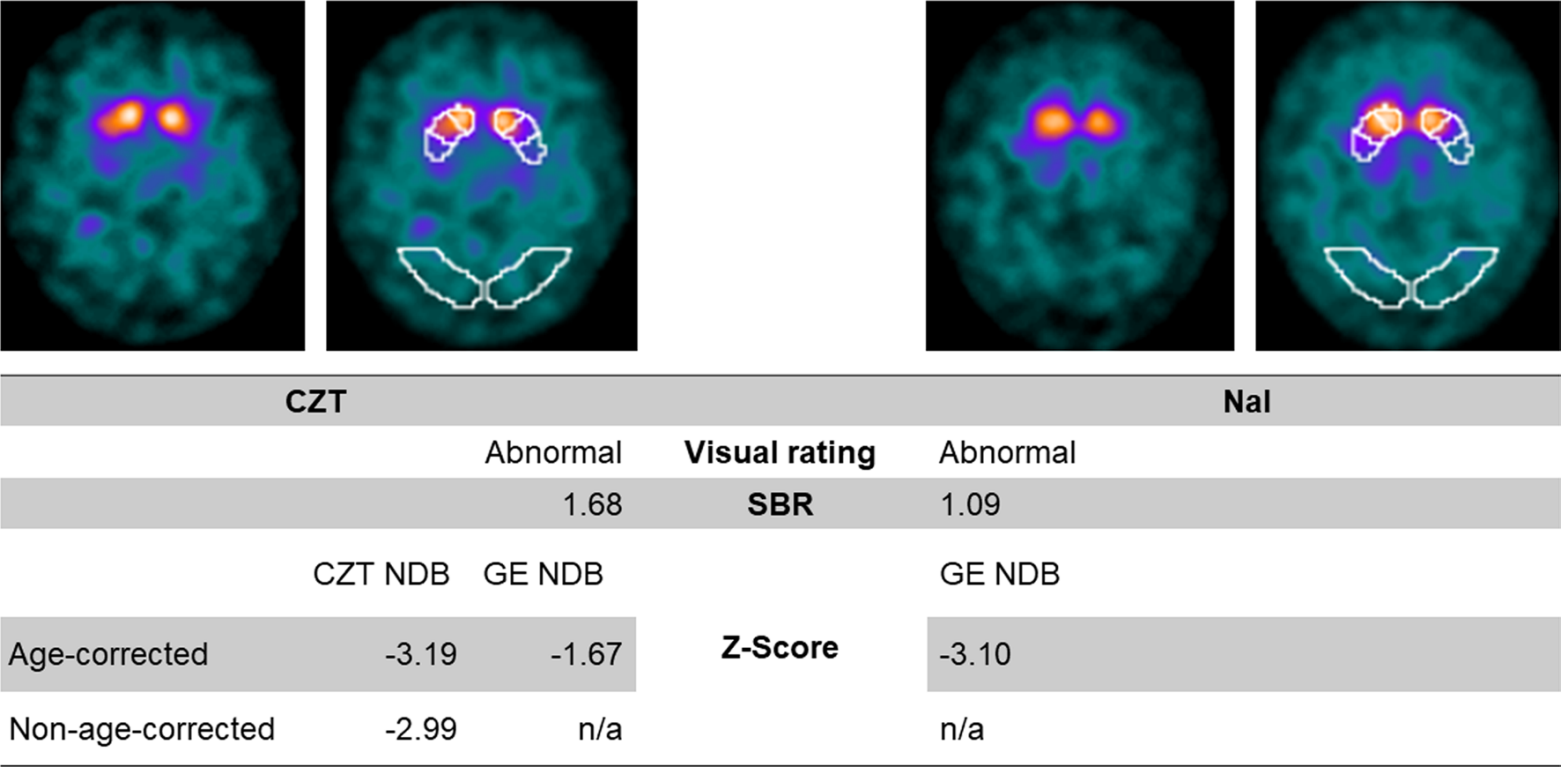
FP-CIT SPECT images of a 65-year-old male first acquired on a NaI (right) and directly afterward on a CZT (left) system. The subject was visually rated as a neurodegenerative PS. For the CZT data, z-scores calculated with the CZT NDB corresponded with the visual assessment, whereas the utilization of the GE NDB resulted in discordance. Semiquantitative results of the NaI system were in line with the visual rating
